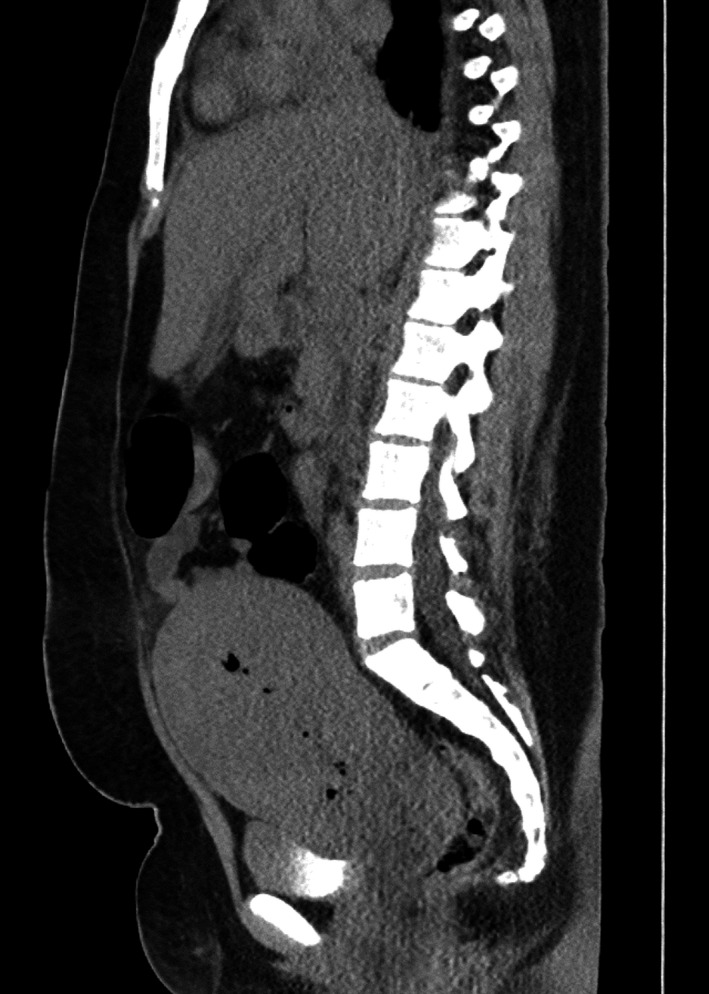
CT abdomen and pelvis, sagittal view. HMG (28 cm), SMG (17.5 cm), hepatic steatosis, small/trace amounts of ascites, enlarged uterus, and tiny pockets of air in the endometrial canal
